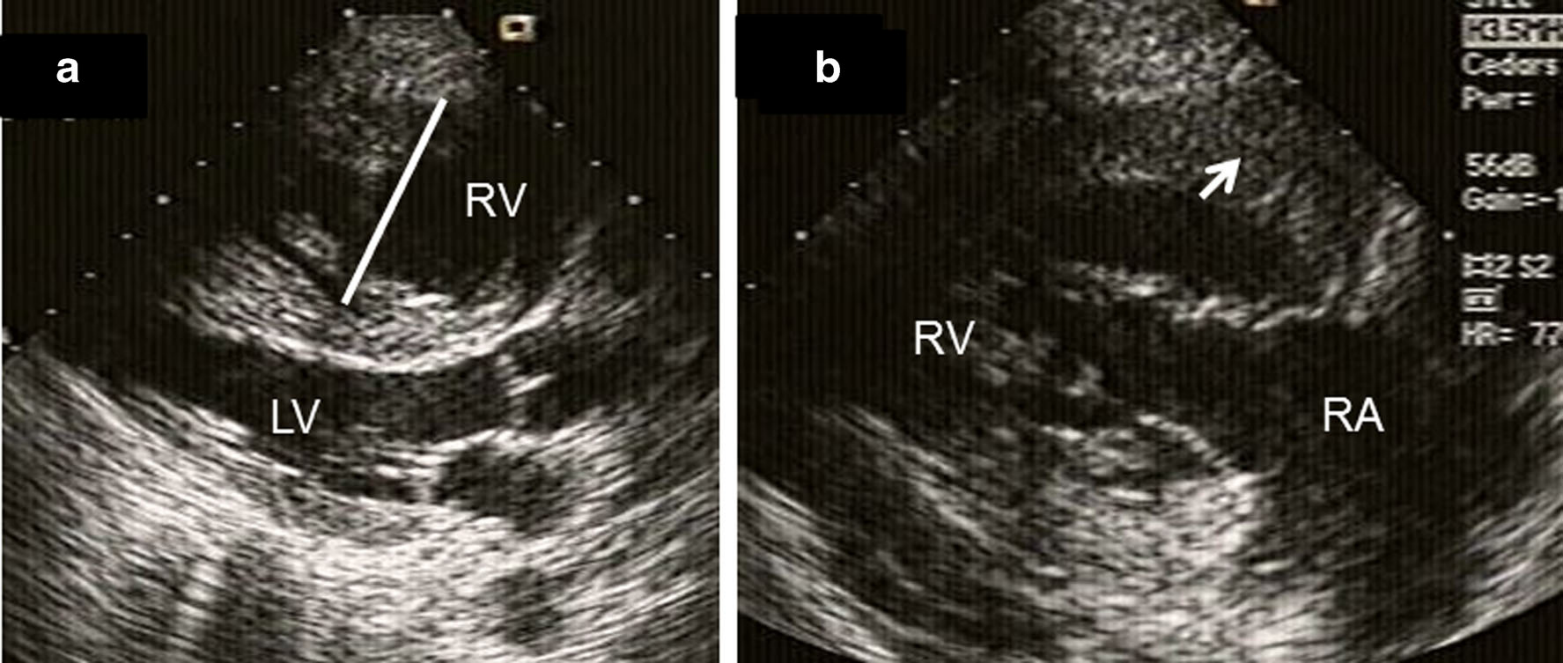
A 20-year-old Hispanic G_1_P_0_ was transferred with a diagnosis of primary pulmonary hypertension at 27-week gestation. There was evidence of polycythemia (Hgb 16 g, Hct 48 %), finger clubbing, and central cyanosis. Pulmonary artery pressure was 90 mm Hg and reduced to 80 mm Hg with inhaled nitric oxide. a Parasternal long-axis view showing severe right ventricular enlargement (*white line*), a small left ventricular cavity, and paradoxical IVS motion. b RV inflow view showing marked right ventricular hypertrophy (*white arrow*). *LV* left ventricle, *RA* right atrium, *RV* right ventricle
